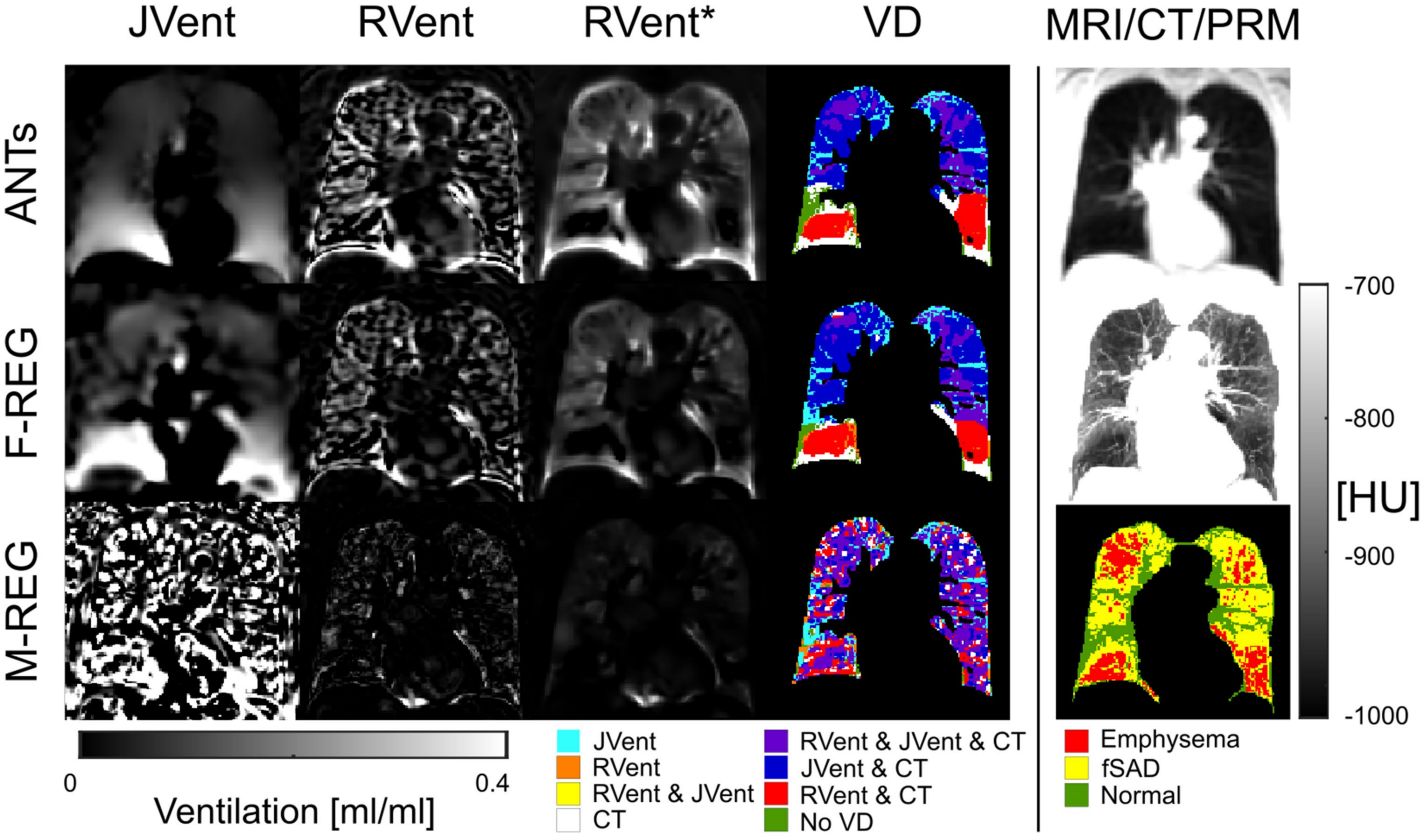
RVent without and with additional filtering (*) in the second and third columns in comparison to Jvent (first column) for a female COPD (Gold IV) patient (age = 70). Rows show the three registration variants. The last column shows the expiration MR image for anatomical reference, CT in expiration, and parametric response mapping (PRM). The fourth column demonstrates the alignment of the respective ventilation defects derived from RVent* and JVent to PRM. JVent and RVent showed PRM-aligned VD in the upper lung. RVent was also aligned in the lower parts. ANTs: advanced normalization tools, F-Reg: Forsberg registration, M-Reg: Matlab registration, RVent: regional ventilation, RVent*: filtered regional ventilation, JVent: Jacobian determinant ventilation, PRM: parametric response mapping, fSAD: functional small airway disease, VD: ventilation defect, COPD: chronic obstructive pulmonary disease.
